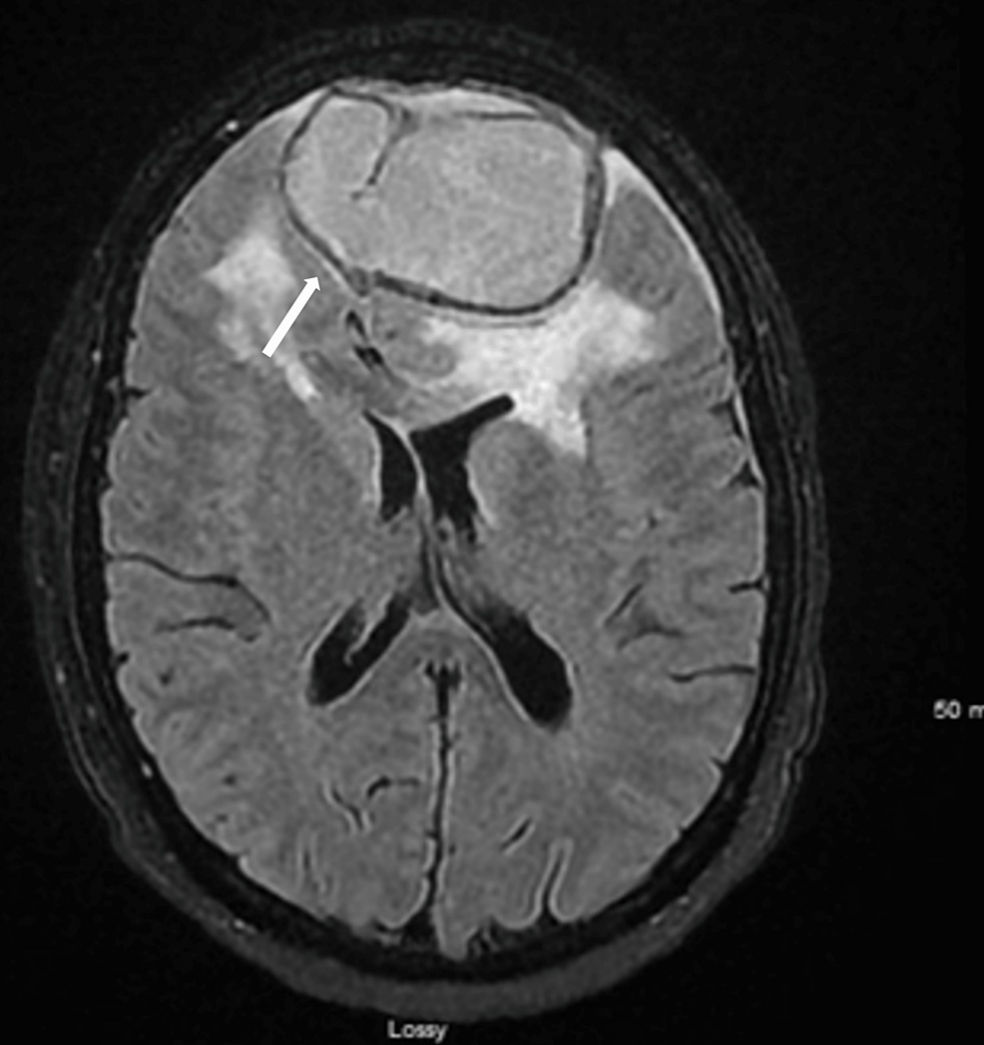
CT scan of the head demonstrating a 5.5 cm intraparenchymal hematoma in the anterior frontal lobe
